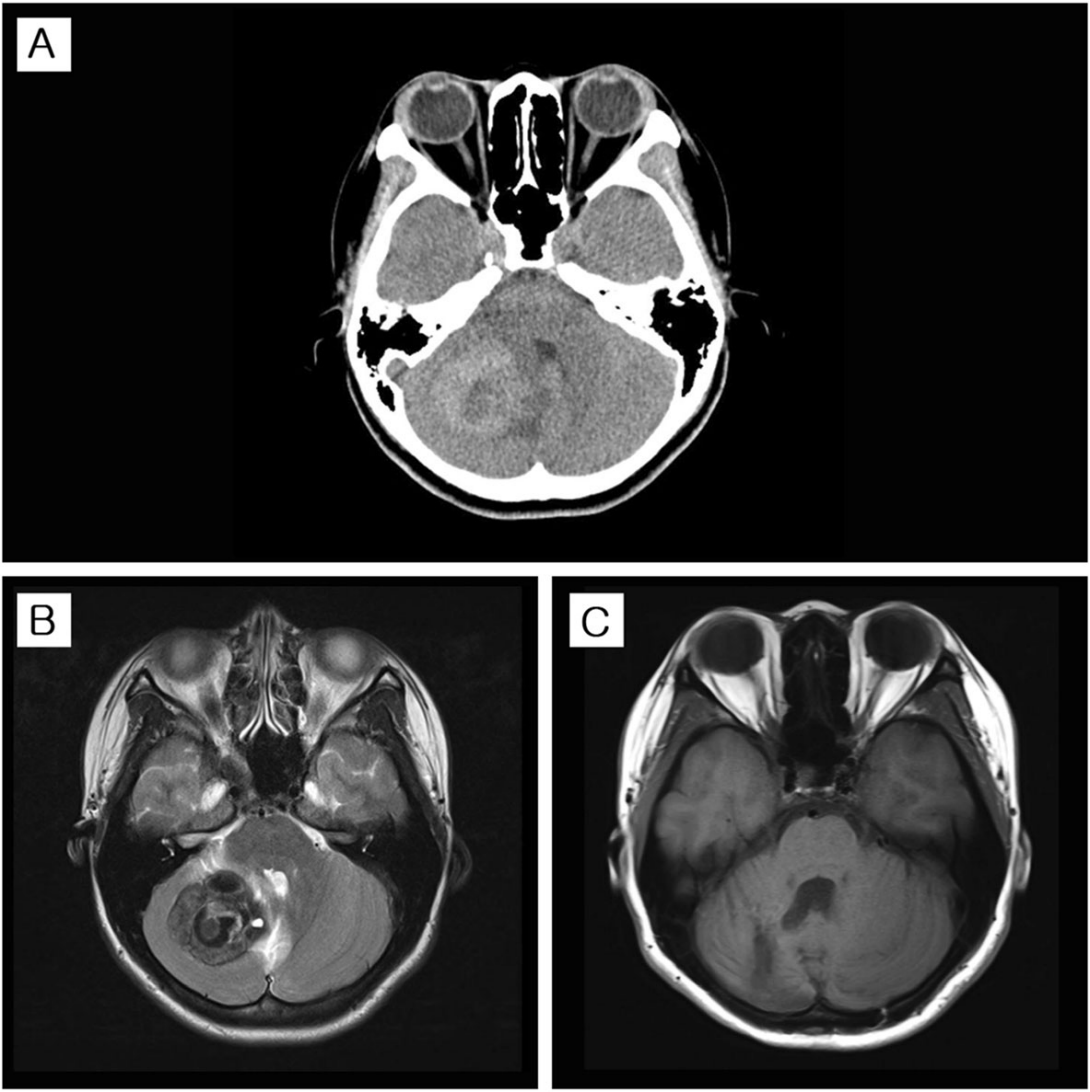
Brain images. Axial CT scan at the onset of the symptoms demonstrated a hemorrhagic mass in the right cerebellar hemisphere and the fourth ventricle compressed by the mass (a). Axial T2-weighted MRI demonstrated heterogeneous low signal intensity of the mass (44 × 37 mm) with high signal intensity in the periphery (b). Axial T1-weighted MRI at 15-month follow-up after resection demonstrated a post-operative malacic lesion in the right cerebellar hemisphere (c)
